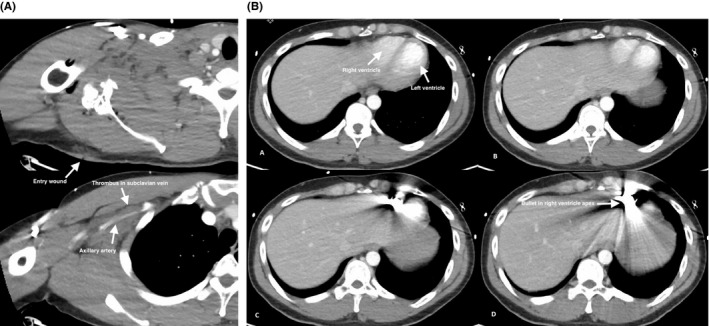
A, Computed tomography scan showing the right shoulder. Upper frame shows posterior gunshot wound and trajectory of the bullet through the glenoid of the scapula. Lower frame shows the axillary artery contrasted and the subclavian vein with a filling defect just anterior to the artery. B, Frames A‐D are sequential cuts, cephalad to caudad, showing the right and left ventricles clearly with a bullet in the right ventricle apex
